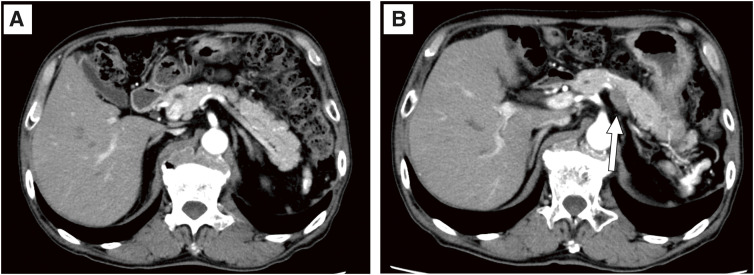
(A) No lymph node metastasis near the splenic artery or pancreas was detected prior to the initial surgery. (B) Two months after the initial surgery, CT revealed an enlarged lymph node measuring 30 × 20 mm adjacent to the splenic artery and pancreas (white arrow). CT, computed tomography
